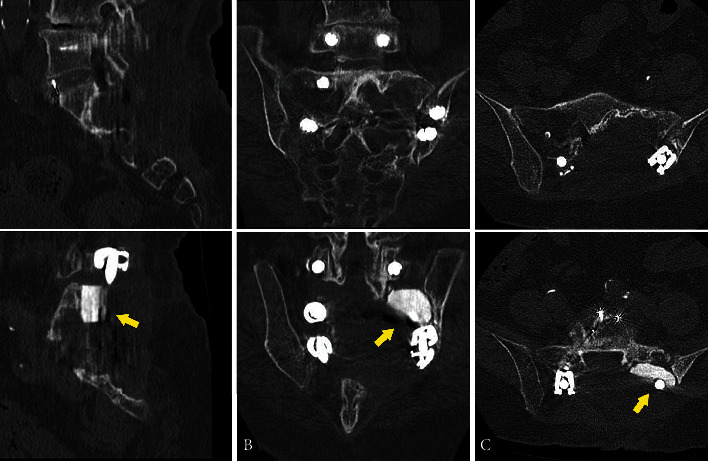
As of 10 months after the surgery, computed tomography shows no recurrence of the tumor and no dislocation of the implants including the *β*-tricalcium phosphate (yellow arrows): (A) sagittal; (B) coronal; (C) axial.
